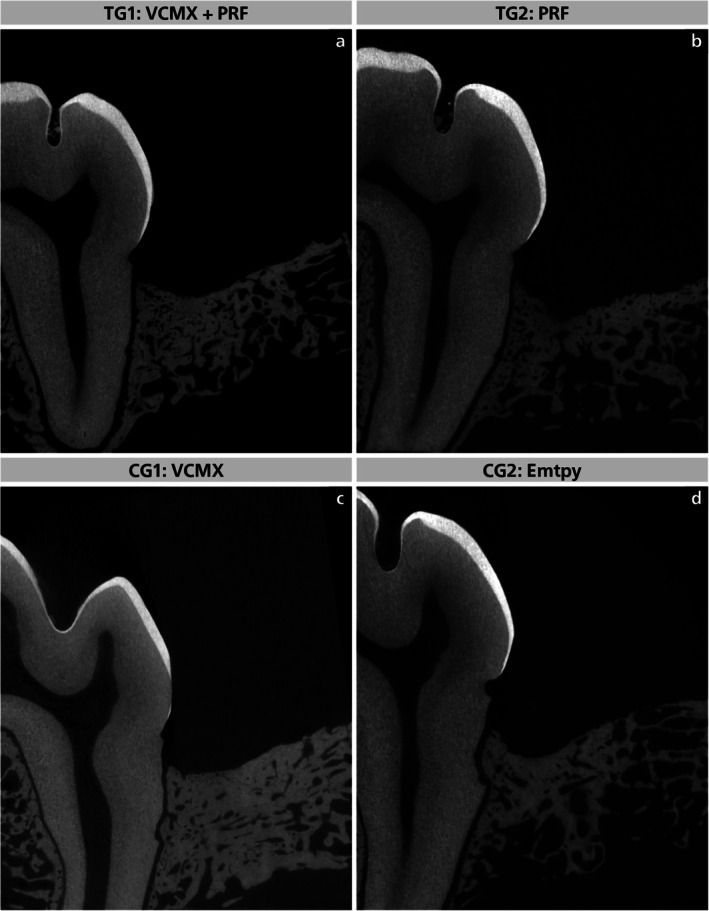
Representative micro‐CT images of TG1 (a), TG2 (b), CG1 (c) and CG2 (d).
